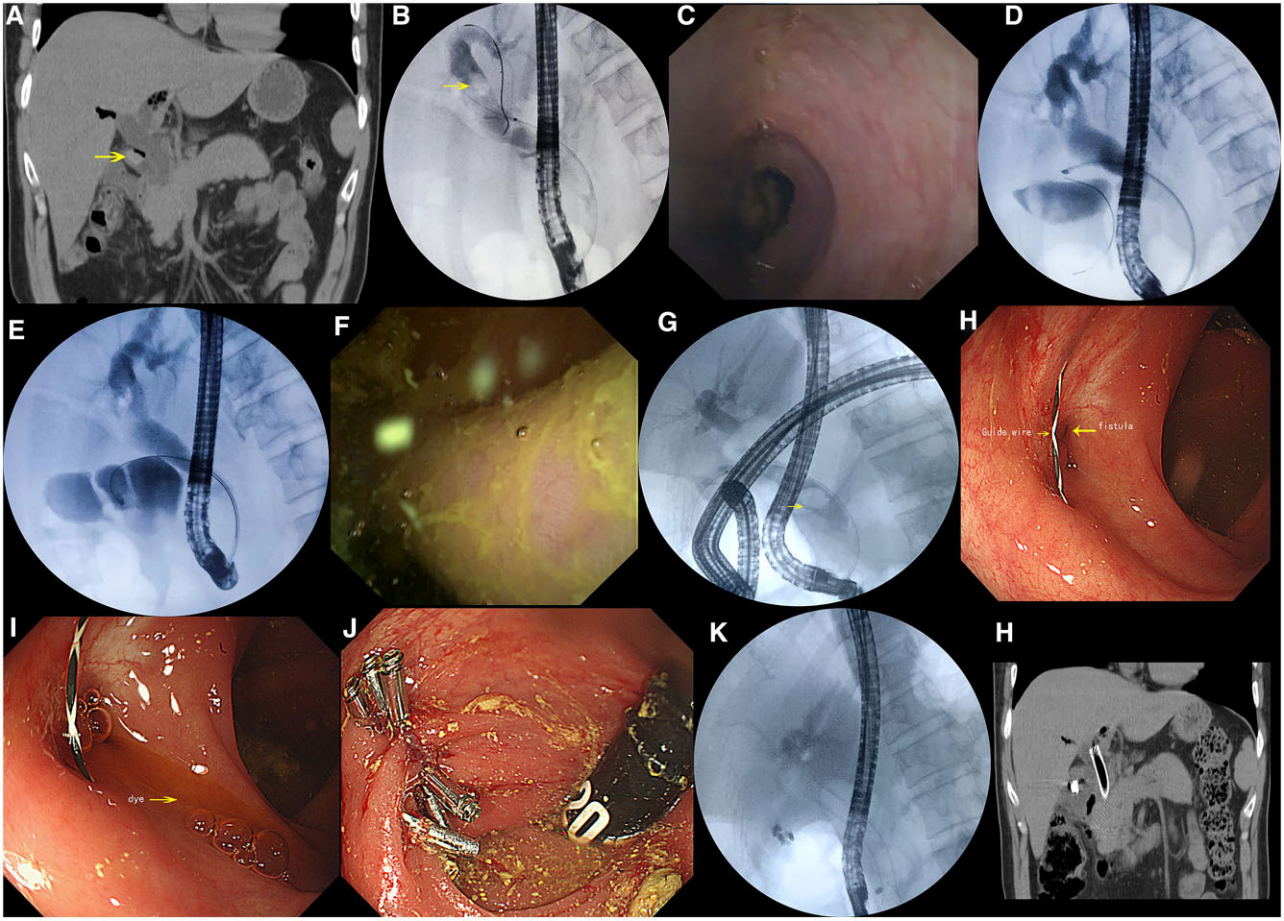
Clinical data of the patient with biliary-colonic fistula. (A) Abdominal computed tomography (CT) showed residual gallbladder neck-duct stone (yellow arrow). (B) Endoscopic retrograde cholangiopancreatography (ERCP) identified choledocholithiasis (yellow arrow). (C) Gallbladder neck-duct stone as seen using the Choledochoscope System. (D) Fluoroscopic image of the guide wire entering the intestinal cavity. (E) The Choledochoscope System enters the intestinal cavity through the sinus tract. (F) Colonic mucosa as seen using the Choledochoscope System. (G) In the second operation, co-use of duodenoscopy and colonoscopy was used. ERCP showed the reflux substance in the bile duct as the guide wire entered the cavity. (H) Guide wire and fistula of colonic hepatic flexure as seen under colonoscopy. (I) Dye injected into the bile duct flowing out from the colonic fistula. (J) Titanium clips were used to clamp the colonic fistula. (K) Fluoroscopic image of the placed biliary stent after recleaning the bile duct. (L) CT examination to confirm biliary stent position.
